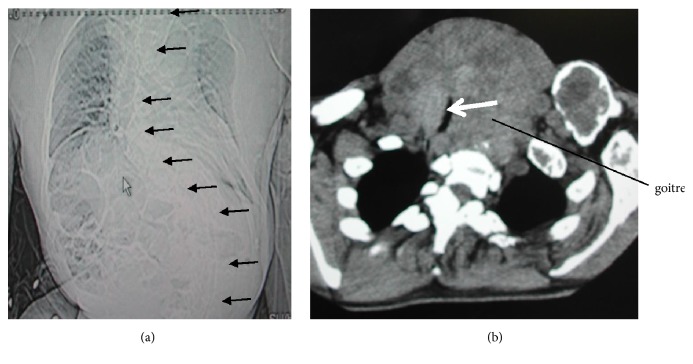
Preoperative CT scan. (a) Lateral curvature of the spine. (b) Narrowest portion of the trachea was 4 mm in diameter in lying down.
